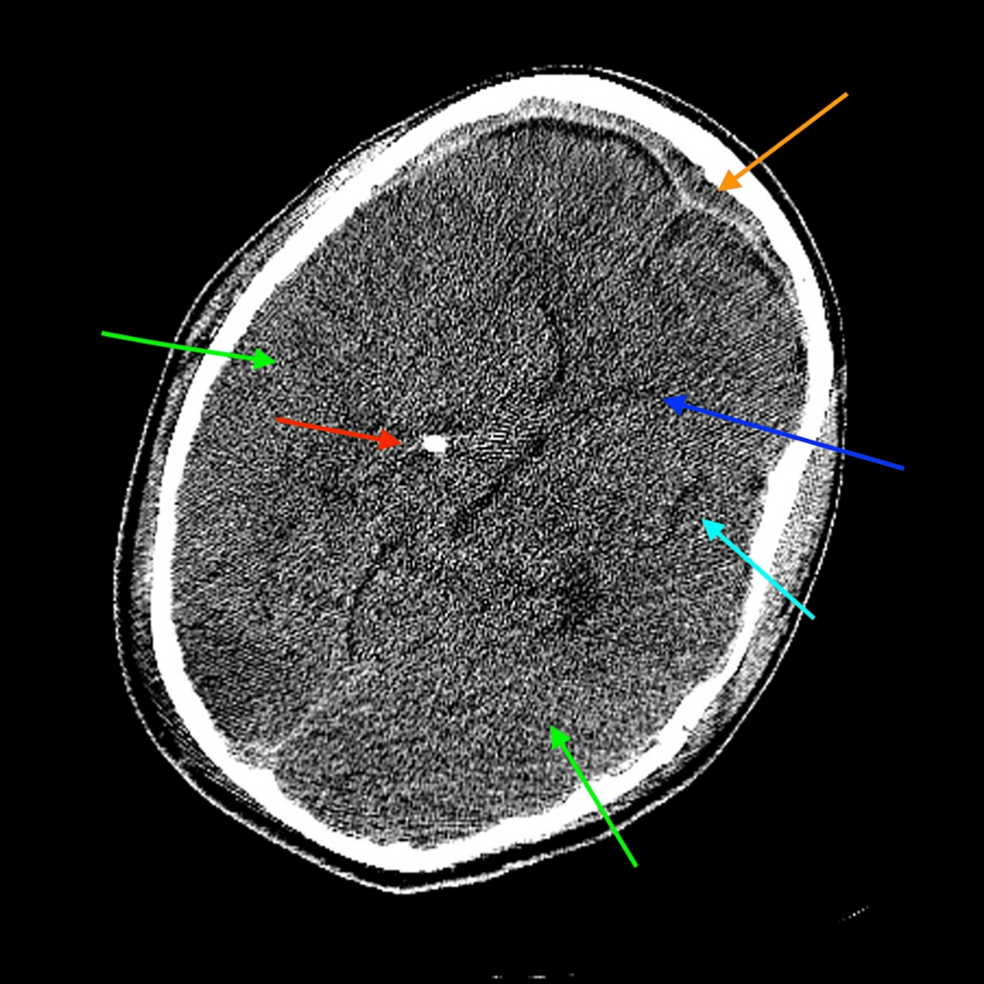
Axial reformat non-contrast CT of the brain at the level of the basal ganglia. Axial reformat non-contrast CT of the brain at the level of the basal ganglia demonstrating frontal and right parietal epidural blood products (orange arrow), diffuse reactive cerebral edema with resultant mass effect, and effacement of the lateral ventricles (dark blue arrow), basal cistern, and sulci (light blue arrow), as well a loss of the gray-white matter differentiation (green arrows). A partially visualized ventriculostomy catheter is present adjacent to the third ventricle (red arrow). CT, computed tomography
